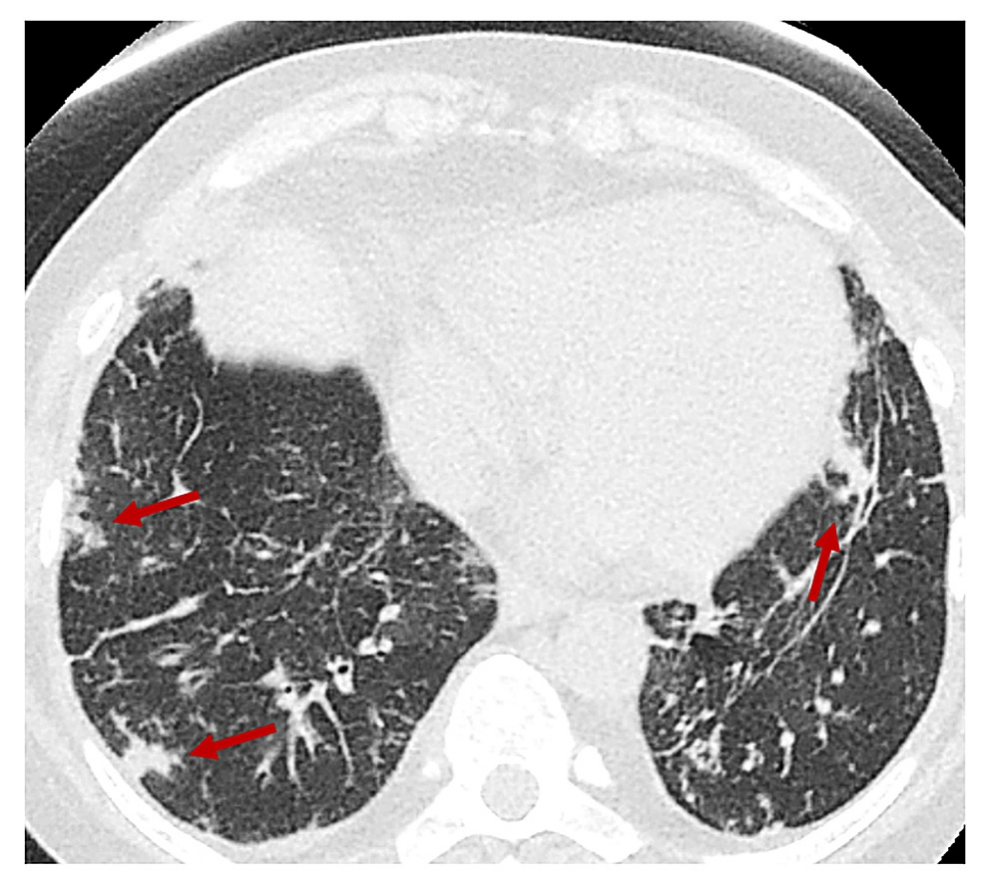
Chest CT without contrast at admission showing multiple areas of nodularity (red arrows) revealing possible septic embolic disease.
